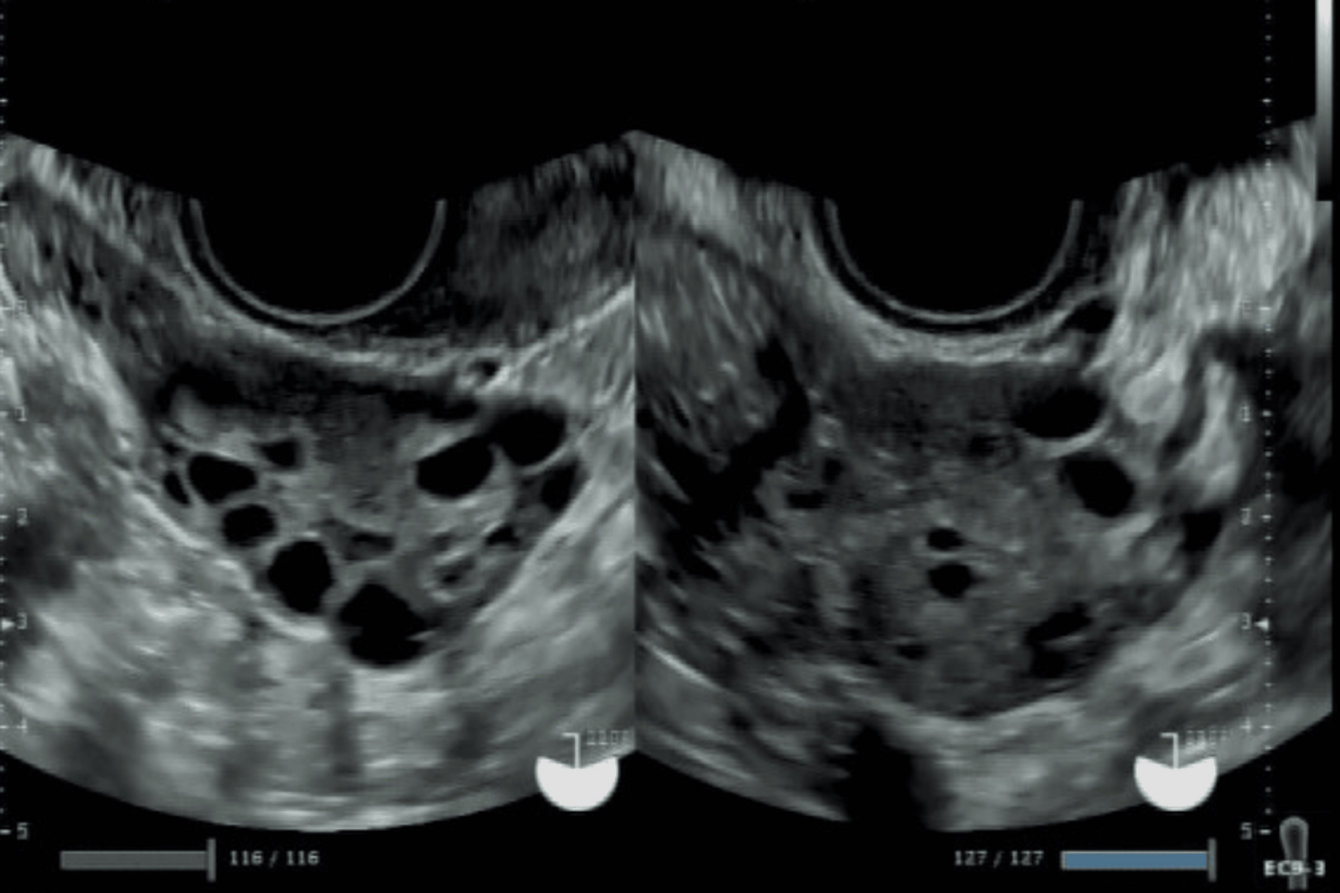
Transvaginal ultrasonography showing polycystic ovary morphology in both ovaries
